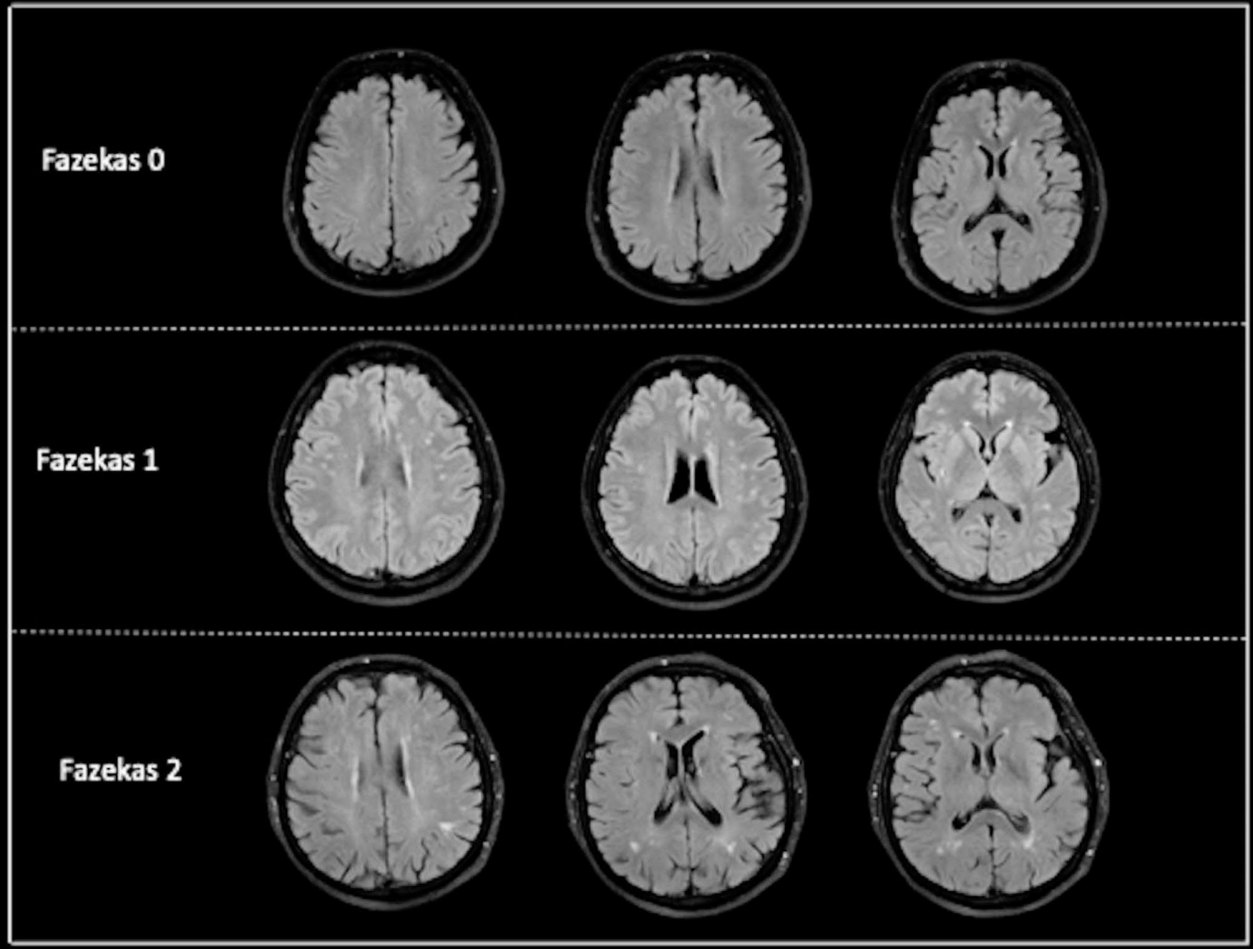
Fazekas grading on brain MRI
